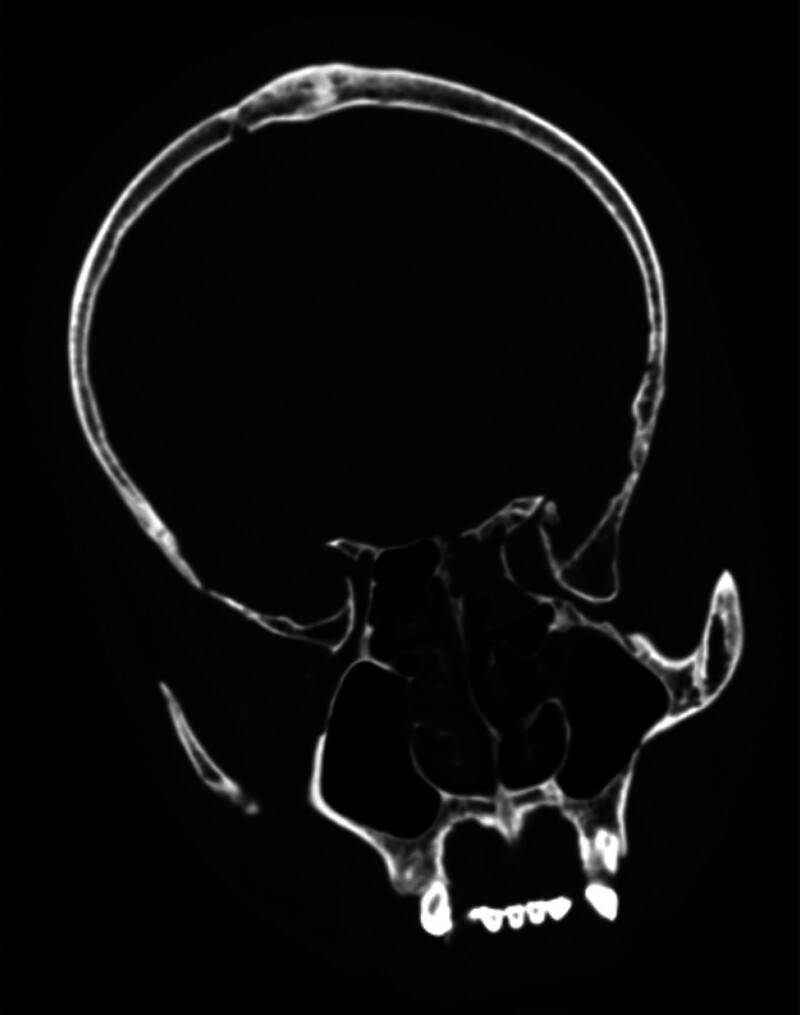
On the CT image of the patient on September 11th, the localization examination of CSF rhinorrhea showed that the bone on the top wall of the right sphenoid sinus was discontinuous, and there were localized bone defects and liquid low-density shadows in the right sphenoid sinus. CSF = cerebrospinal fluid, CT = computed tomography.
